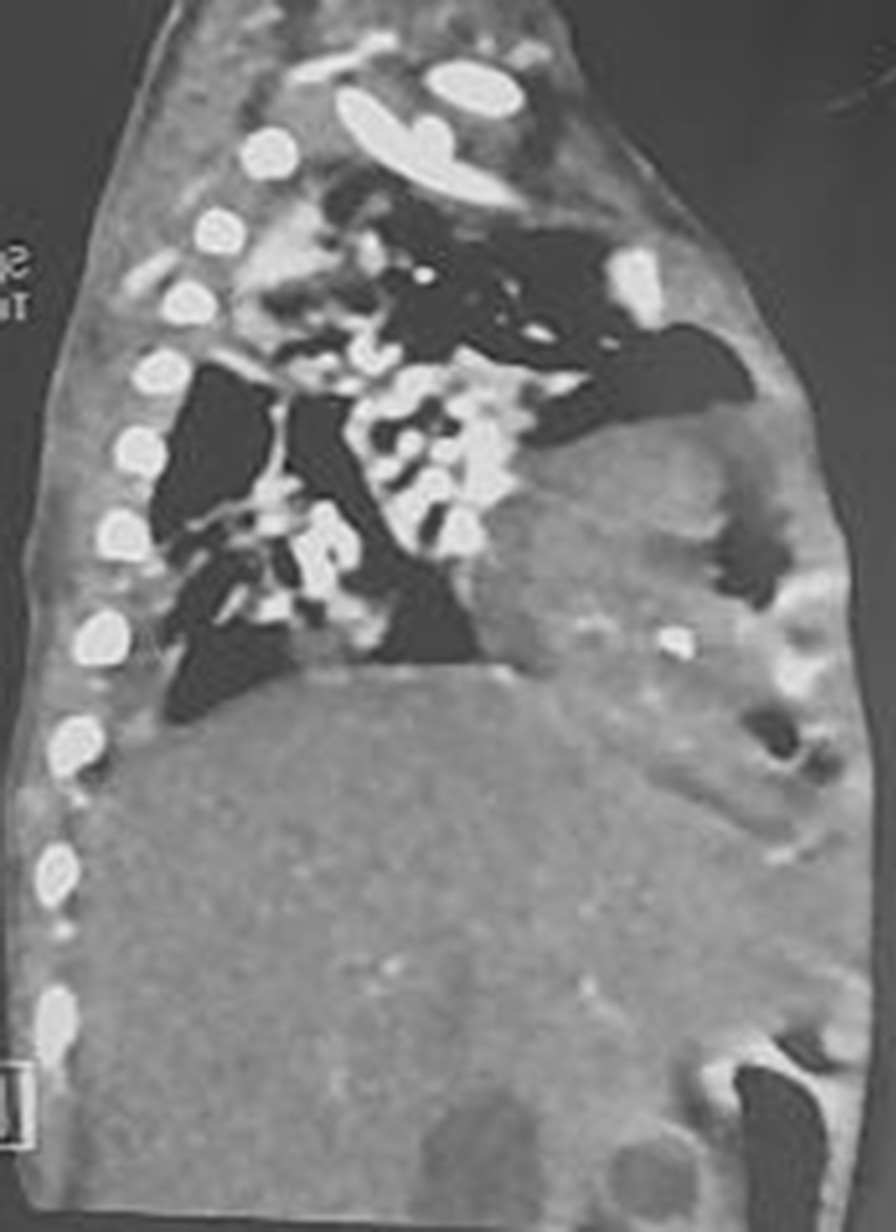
Lateral view of the thorax on contrast-enhanced computed tomography shows elevation at the anterior portion of right hemidiaphragm containing abdominal viscera
